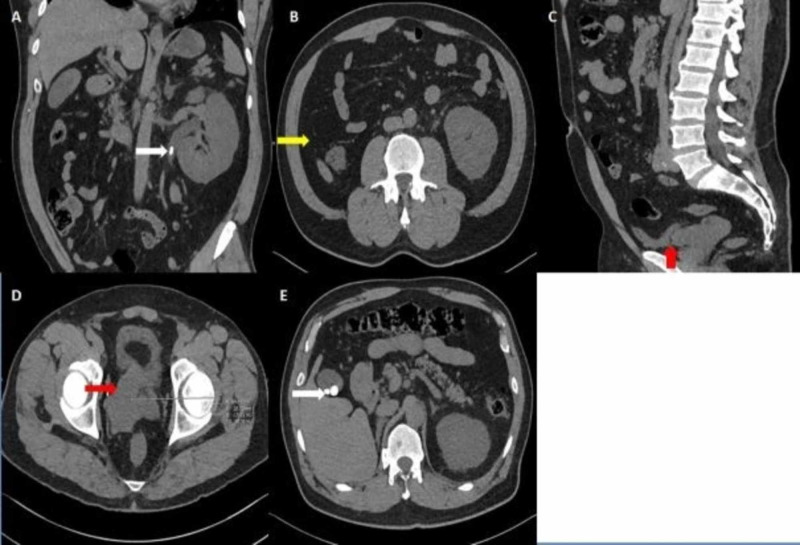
Unenhanced CT of the abdomen and pelvis An oblique coronal view demonstrates a left upper ureteric obstructing stone, causing dilatation of the proximal ureter and pelvicalyceal system (A). An axial section at the renal level portrays that the right renal fossa is empty, with no renal tissue or surgical materials (B). A sagittal and an axial cut revealing a right retrovesical possibly cystic lesion in the expected anatomic location of the right seminal vesicle (C, D). An axial cut of the upper abdomen exhibiting incidental finding of a few gallbladder stones without surrounding inflammatory changes (E).
